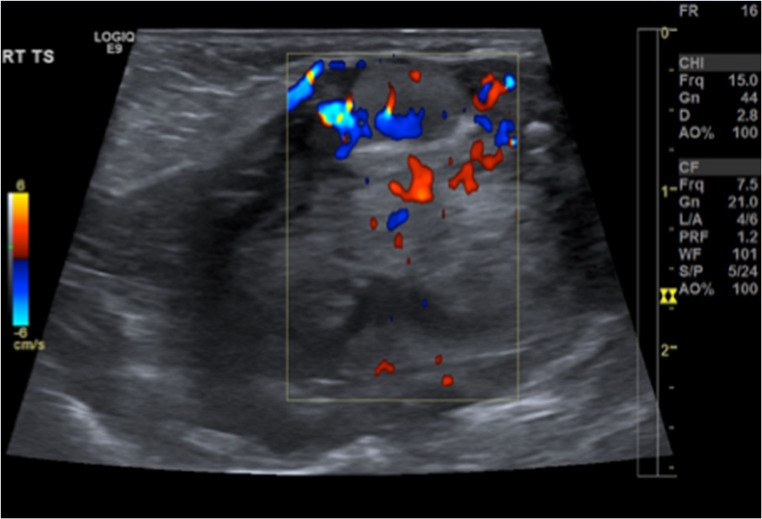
Ultrasound neck with Doppler showing a 6.5 mm x 5.6 mm nodule of thyroid tissue high in the neck above the level of the hyoid cartilage with vascularity on Doppler
